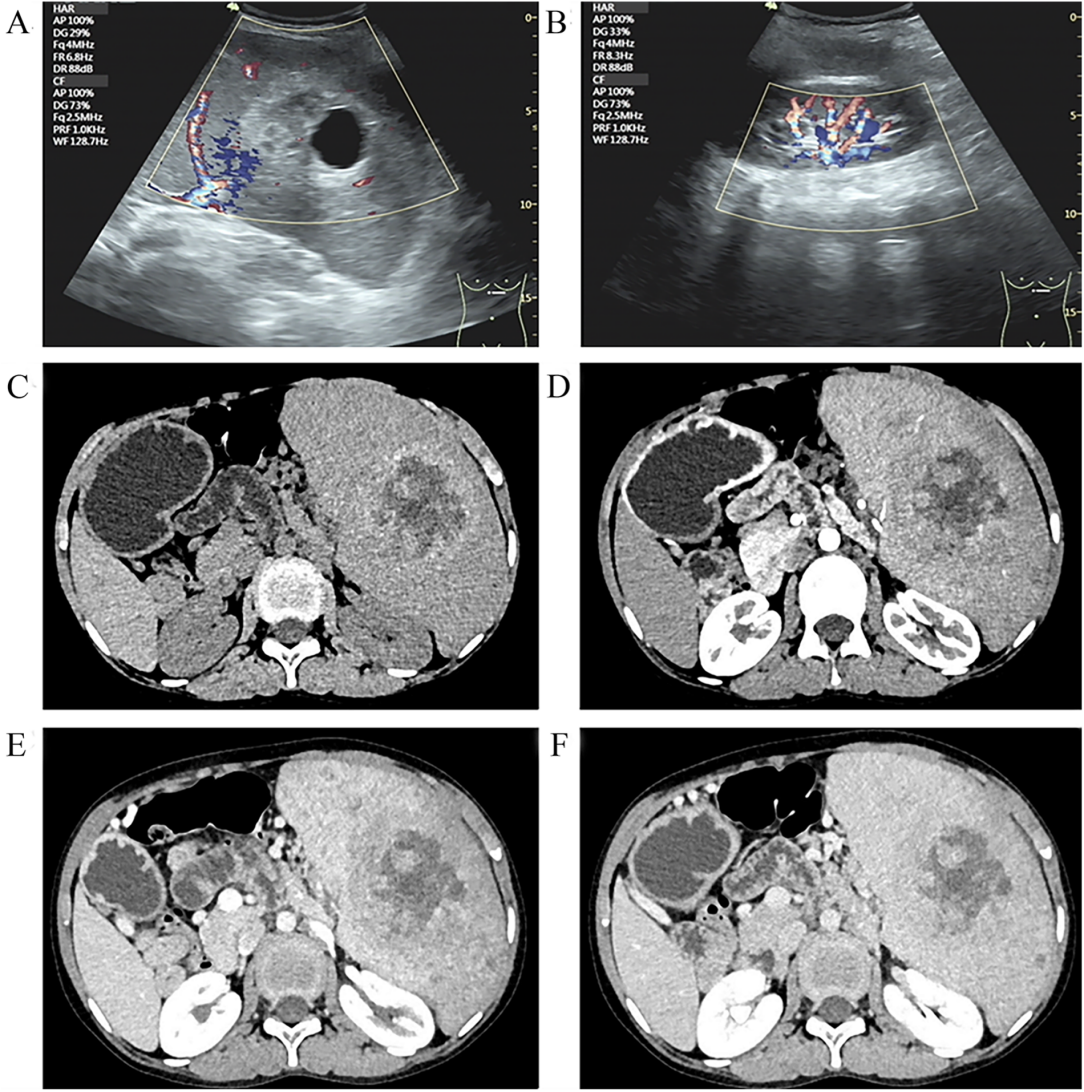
Ultrasound images of LCA (A,B): ultrasound indicated that the internal echoes of the spleen were heterogeneous, measuring approximately 7.4 cm × 6.8 cm. CT images of LCA (C–F) indicated multiple low-density lesions: (C) Non-contrast-enhanced CT showed low-density splenic masses; (D) the arterial phase of contrast-enhanced CT became clearer, though the peripheral enhancement of the tumor remained unobvious; (E) the venous phase of contrast-enhanced CT showed the enhancement degree of the tumor mimicking normal spleen tissue; and (F) the delayed phase of contrast-enhanced CT showed the highest CT value.
